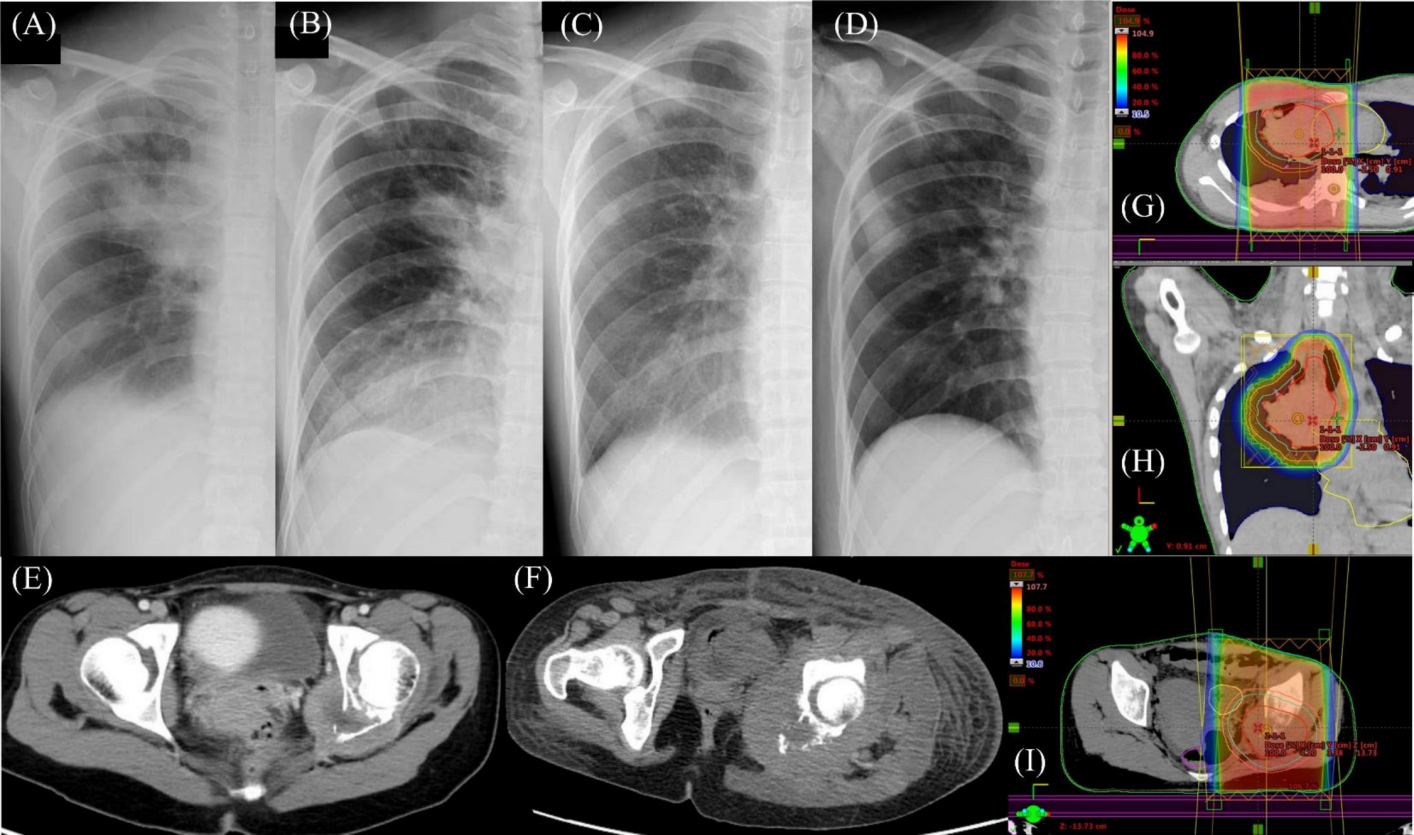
Radiographic course and radiotherapy planning CT findings. (A) Facial oedema and headache due to SVC syndrome improved promptly after thoracic palliative radiotherapy (20 Gy in 5 fractions). (B, C) The right hilar mass showed marked shrinkage at 1 week (B) and sustained reduction at 1 month (C) after thoracic irradiation. (D) At 16 weeks, a new right lateral pulmonary recurrence developed in a region that had received a substantially lower radiation dose than the high‐dose hilar field. (E, F) In contrast, the left pelvic bone metastasis treated with single‐fraction radiotherapy (8 Gy in 1 fraction) showed radiographic progression by 16 weeks, despite transient symptom relief. (G, H) Axial (G) and coronal (H) radiotherapy planning CT images demonstrating the dose distribution for thoracic irradiation, with tumour response localised to the higher‐dose region. (I) Radiotherapy planning CT image for the pelvis showing the dose distribution surrounding the left ischial lesion. Images in panels (B–F) were obtained during the same terminal phase of the disease, allowing direct comparison of radiographic response within and outside the thoracic radiation field and at the pelvic metastatic site.
